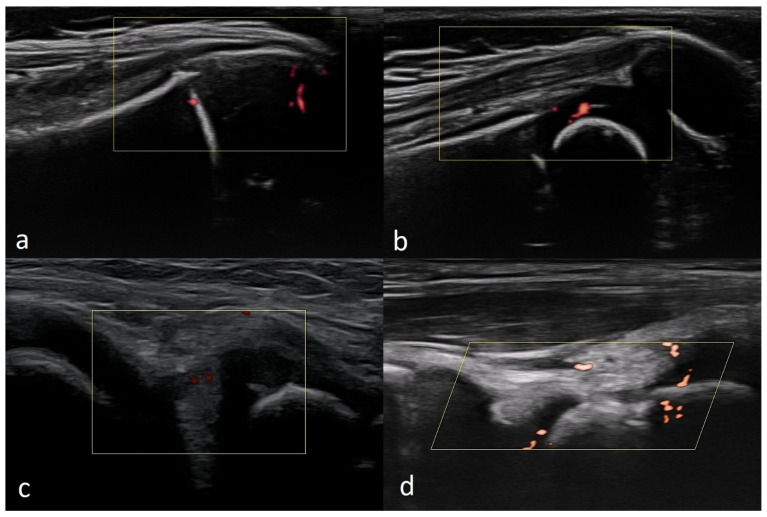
Examples of increased flow in Power Doppler imaging in children, which do not correspond to a pathology. (a) Frontal plane scan from the side—visible vessels within hyaline cartilage of the epiphysis of the femur of a 6-month-old boy. Linear probe 3–19 MHz. (b) Longitudinal scan of the suprapatellar recess of the same child—visible vessels near the growth plate. (c) Longitudinal scan of the posterior-medial part of the knee joint of a 9-year-old girl. Visible flow in vessels in the pericapsular zone of the posterior part of the medial meniscus. Linear probe 3–12 MHz. (d) Longitudinal scan of the anterior recess of the ankle joint of a 5-month-old boy. The visible signals are artifacts from excessive motion. Linear probe 10–25 MHz.
